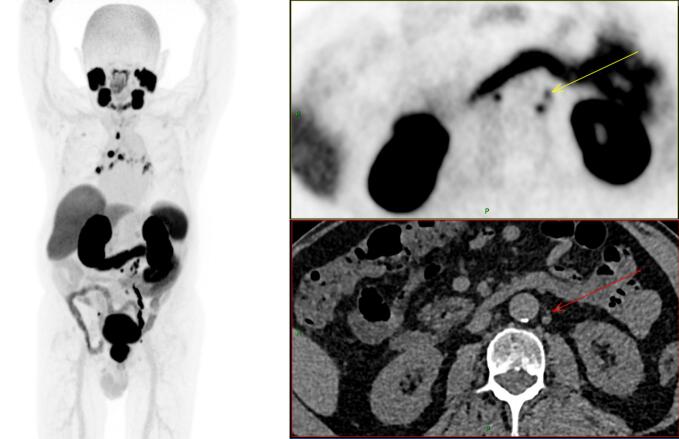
[^68^Ga]Ga-PSMA-11 PET-CT maximum intensity projection, PET and CT transaxial images from a long axial field of view scanner (body mass index 27.5 kg/m^2^, 108 MBq (1.5 MBq/kg), 10 mins static acquisition) in a patient for primary staging of prostate cancer demonstrating diffuse involvement of prostate and extensive PSMA-avid nodal disease in pelvis, retroperitoneal and thoracic nodes. Arrows point at a 2.8 mm PSMA-avid node. Low noise and high sensitivity can be appreciated.
